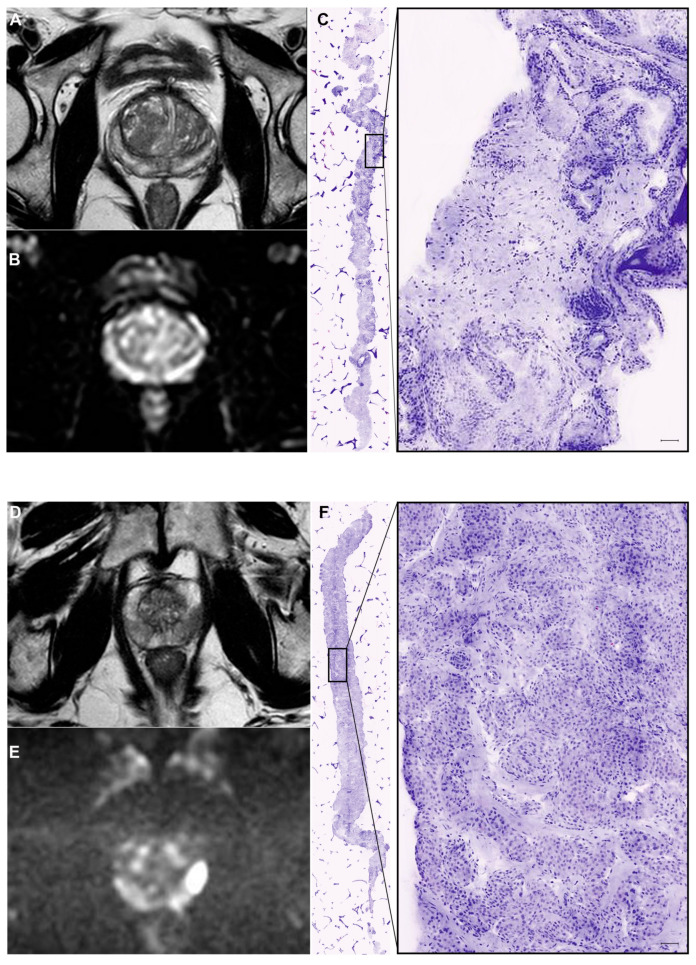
Representative MRI and digital pathology images of two patients included in the study. (A–C) Patient 10: PSA 8.77 ng/mL, Suspicious digitorectal examination (DRE), negative MRI ((A): T2W image; (B): DWI Image), negative digital biopsy (C). (D–F) Patient 5: PSA 5.14 ng/mL, negative DRE, suspicious MRI (PIRADS 4; (D): T2W image; (E): DWI image), positive digital biopsy (F).
